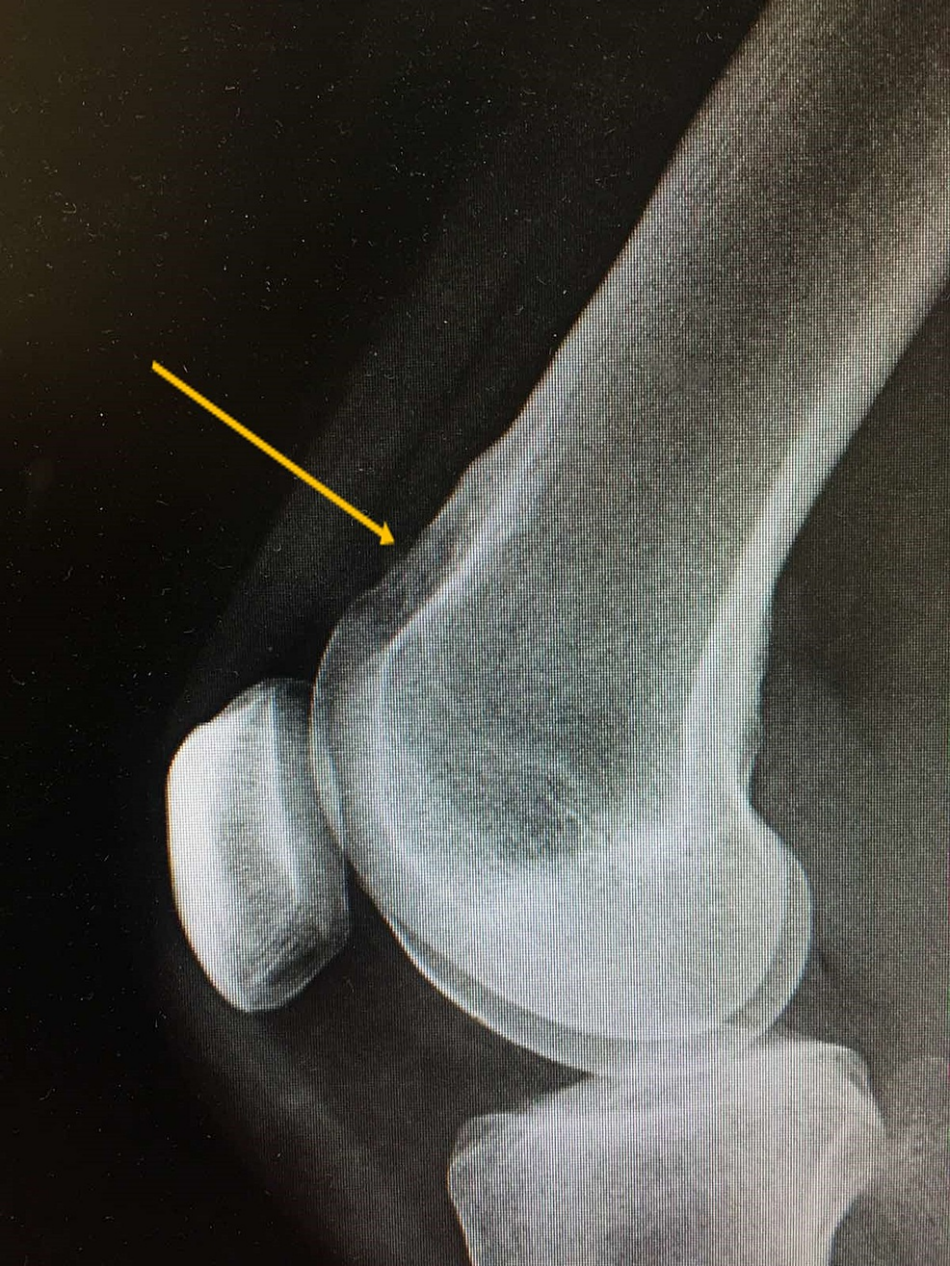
Lateral radiograph of the patient's knee six months postoperatively showing complete removal of the bone lesion (yellow arrow).
